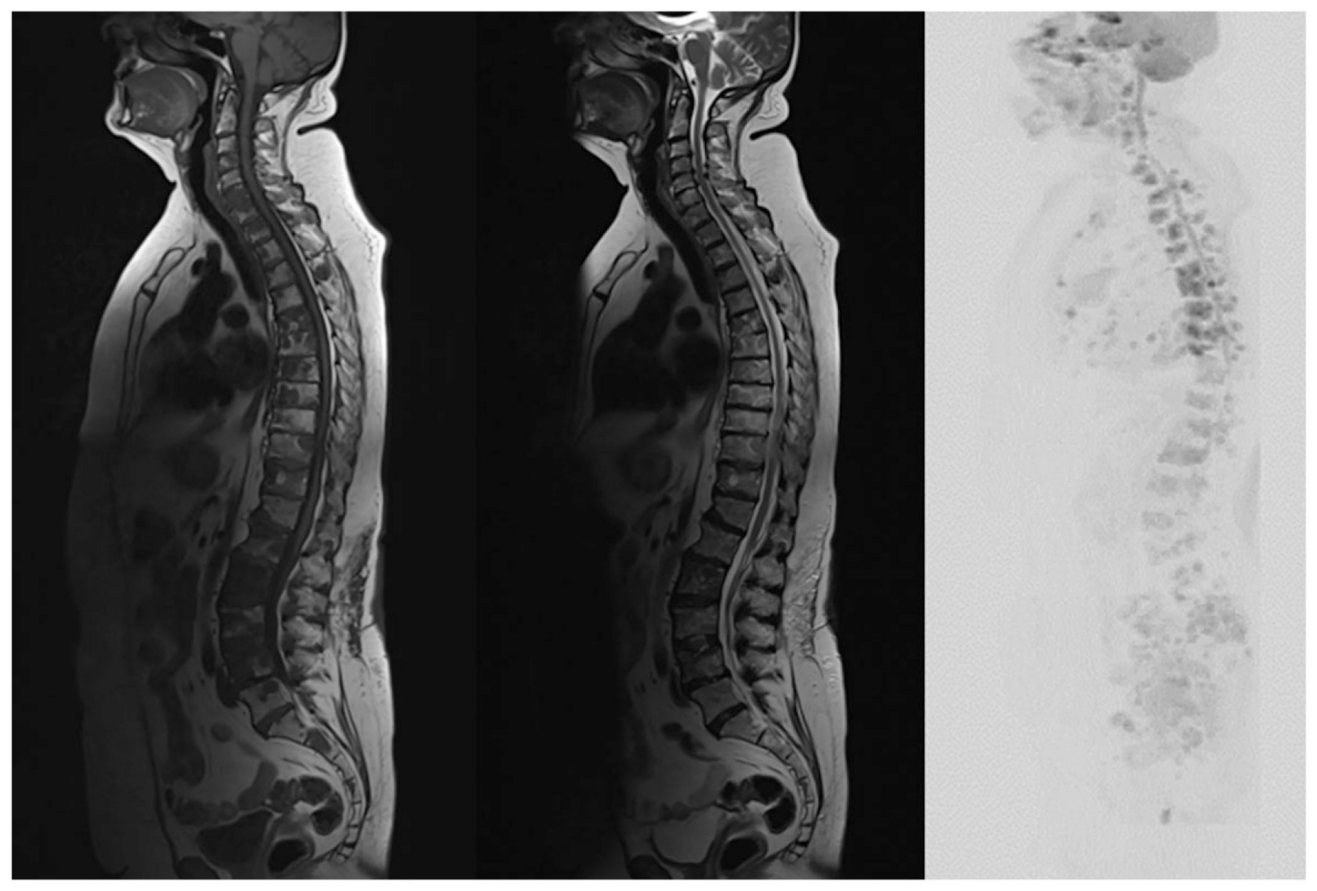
T1-weighted (left), T2-weighted (center), and diffusion-weighted inverted maximum-intensity projection (b800 s/mm^2^) (right) sagittal sequences demonstrating multiple bone metastases in a patient with metastatic breast cancer. The metastases show a low signal on T1-weighted images, a low-to-intermediate signal on T2-weighted images, and an increased signal on diffusion-weighted imaging.
